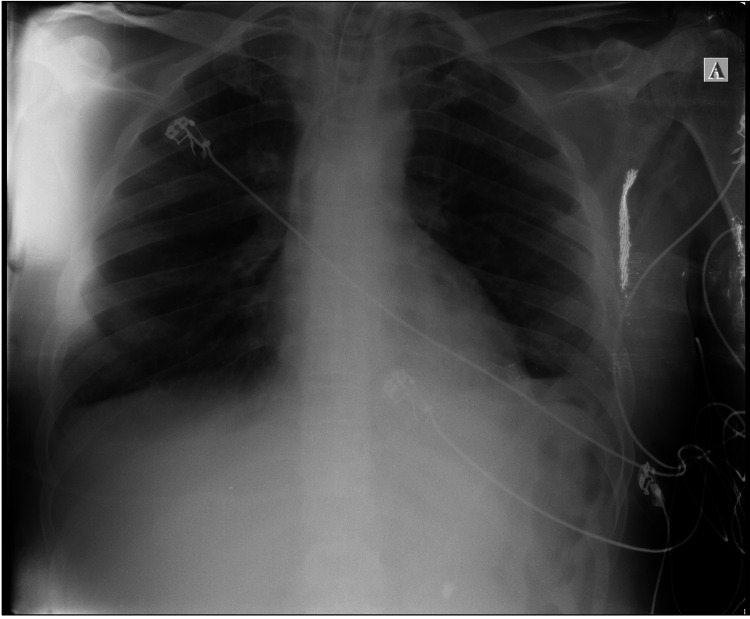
Final X-ray taken before discharge. The pleural effusion seen in the previous X-ray has resolved.
